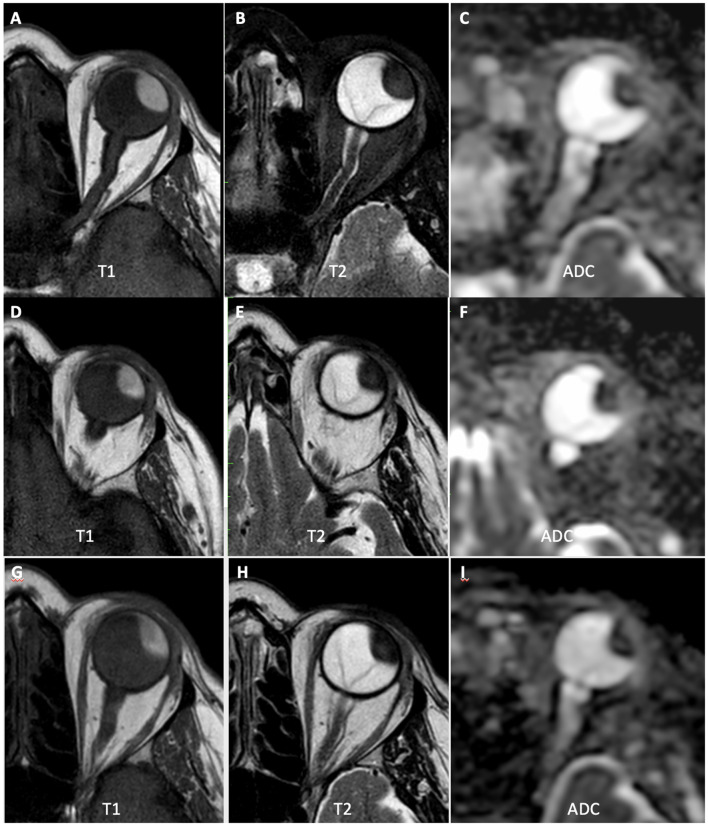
A 66-year-old patient with choroidal melanoma in the left eye (upper temporal quadrant, measuring 15 × 14 × 9 mm), was treated with brachytherapy. (A–C) MR1 showing tumor with high signal at T1-weighted images, low signal at T2-weighted images, and restricted diffusion on the ADC map (mean ADC: 0.90 × 10^−3^ mm^2^/s). (D–F) MR2 showing that the tumor had similar morphological characteristics and dimensions, but with a slight increase in ADC values compared to the initial examination (mean ADC: 0.97 × 10-3 mm2/s). (G–I) MR3 showing a slight reduction in tumor dimensions, with further increase in ADC values (mean ADC: 1.20 × 10^−3^ mm^2^/s).
